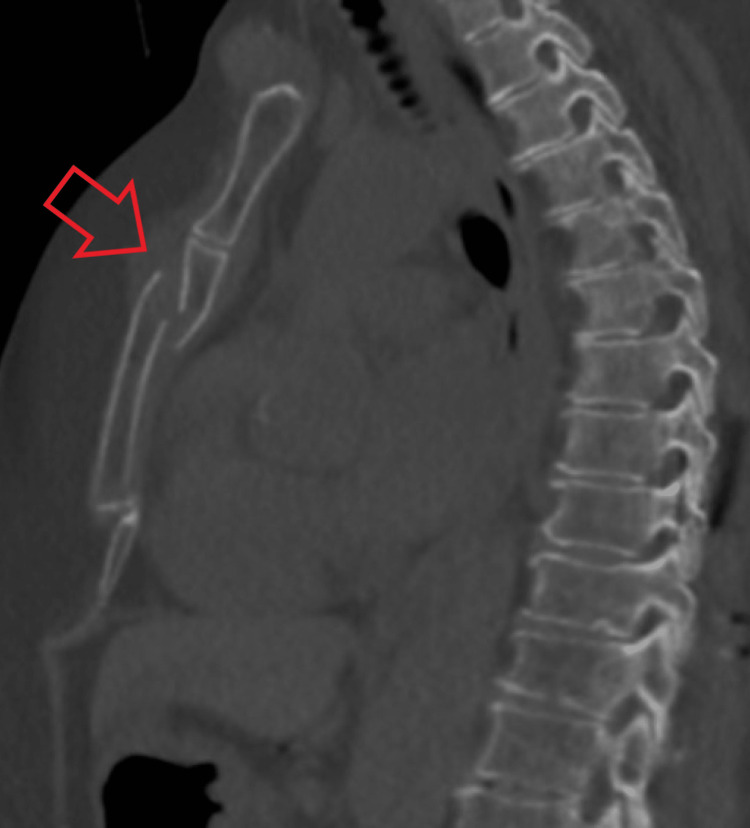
Sagittal CT image demonstrating posteriorly displaced and inferiorly distracted sternal fracture (bone window). The red arrow indicates the fractured sternum.
